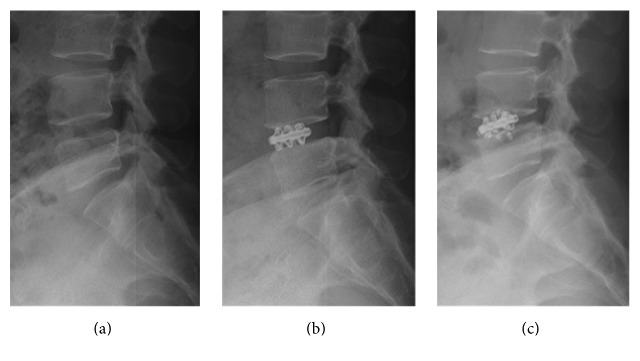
A 50-year-old female patient (patient number: 3 in the tables) with DDD at the L4-5 level. Lateral standing X-rays showing (a) preoperative, (b) early postoperative, and (c) final follow-up X-rays including standing lateral neutral views taken at 32 months after the surgery. It can be seen that the limbs of the implant are broken and there is a 2.5 mm reduction in DH at the final follow-up examination compared to the early postoperative period. The patient's VAS-B, VAS-L, and ODI scores were 4, 2.5, 31.1, respectively, at the final follow-up visit. The patient rated her result as “fair.”
